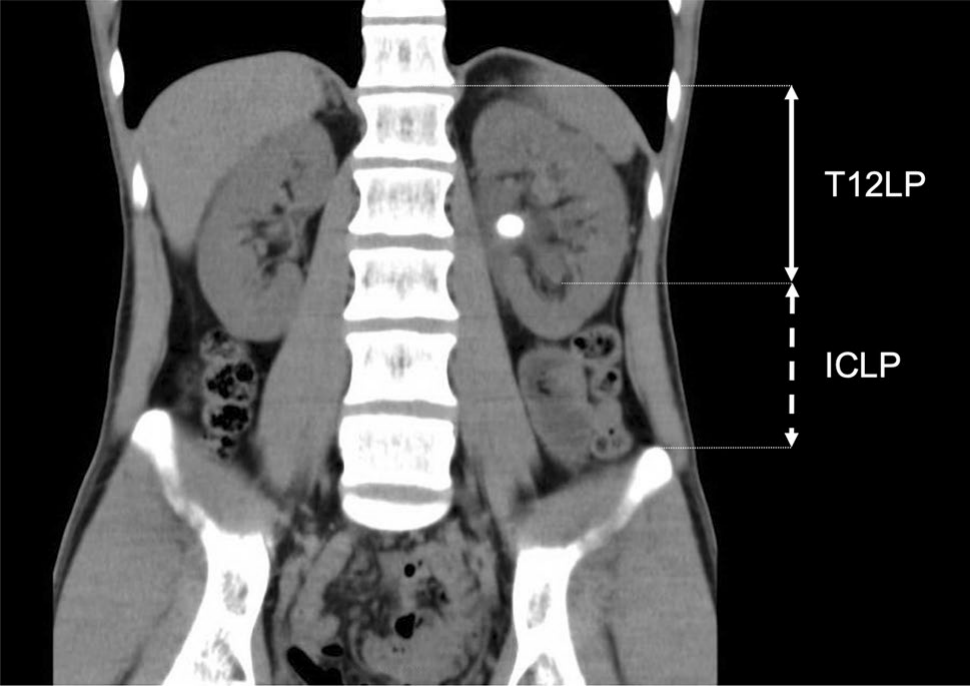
Minimal distance between T12 vertebra and lower calyx (T12LP) and between lower calyx and iliac crest (ICLP) measured on coronal CT images
